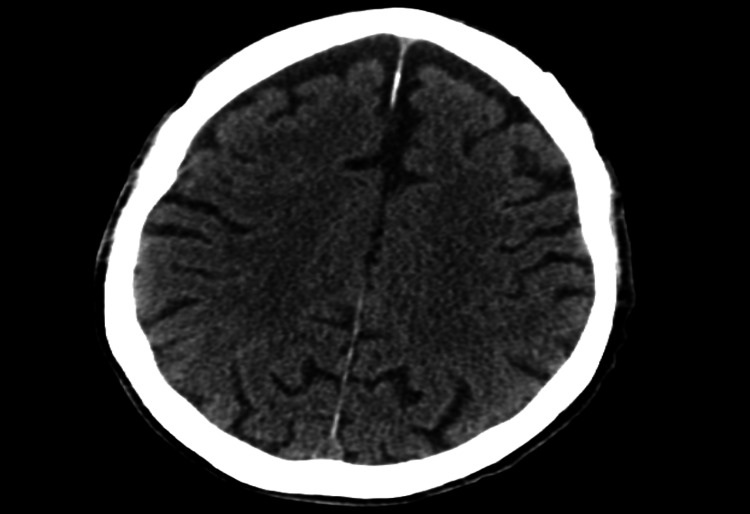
CT head without contrast showing no intracranial hemorrhage or acute infarct, primarily in the left precentral gyrus. CT, computed tomography
